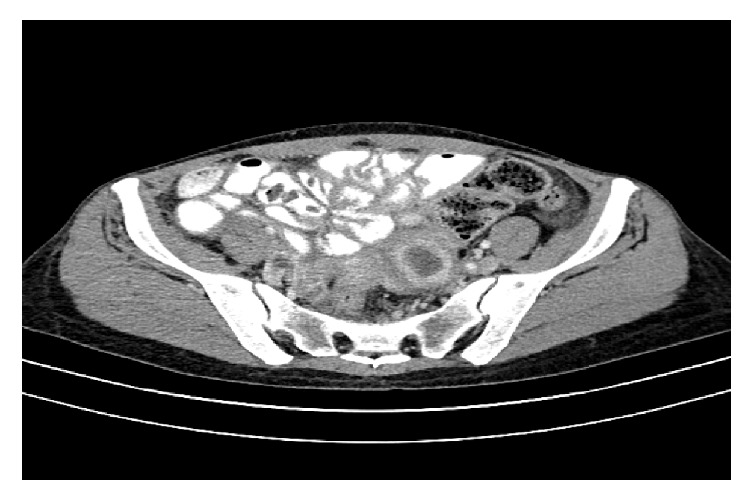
CT of pelvis demonstrating reduction of adnexal mass and decompressed bowel loops at 1 year of treatment.
